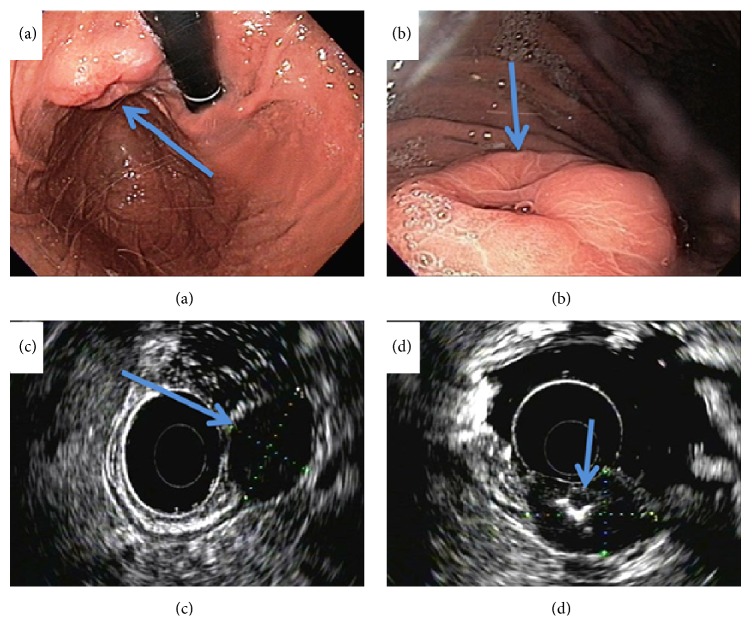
Esophagogastroduodenoscopy (EGD) and endoscopic ultrasound (EUS) of the gastric mass. (a)-(b) EGD shows the 3.5 × 2.5 × 1.5 cm subepithelial gastric mass with central umbilication (blue arrow). (c)-(d) EUS shows the gastric mass is hypoechoic (blue arrow).
